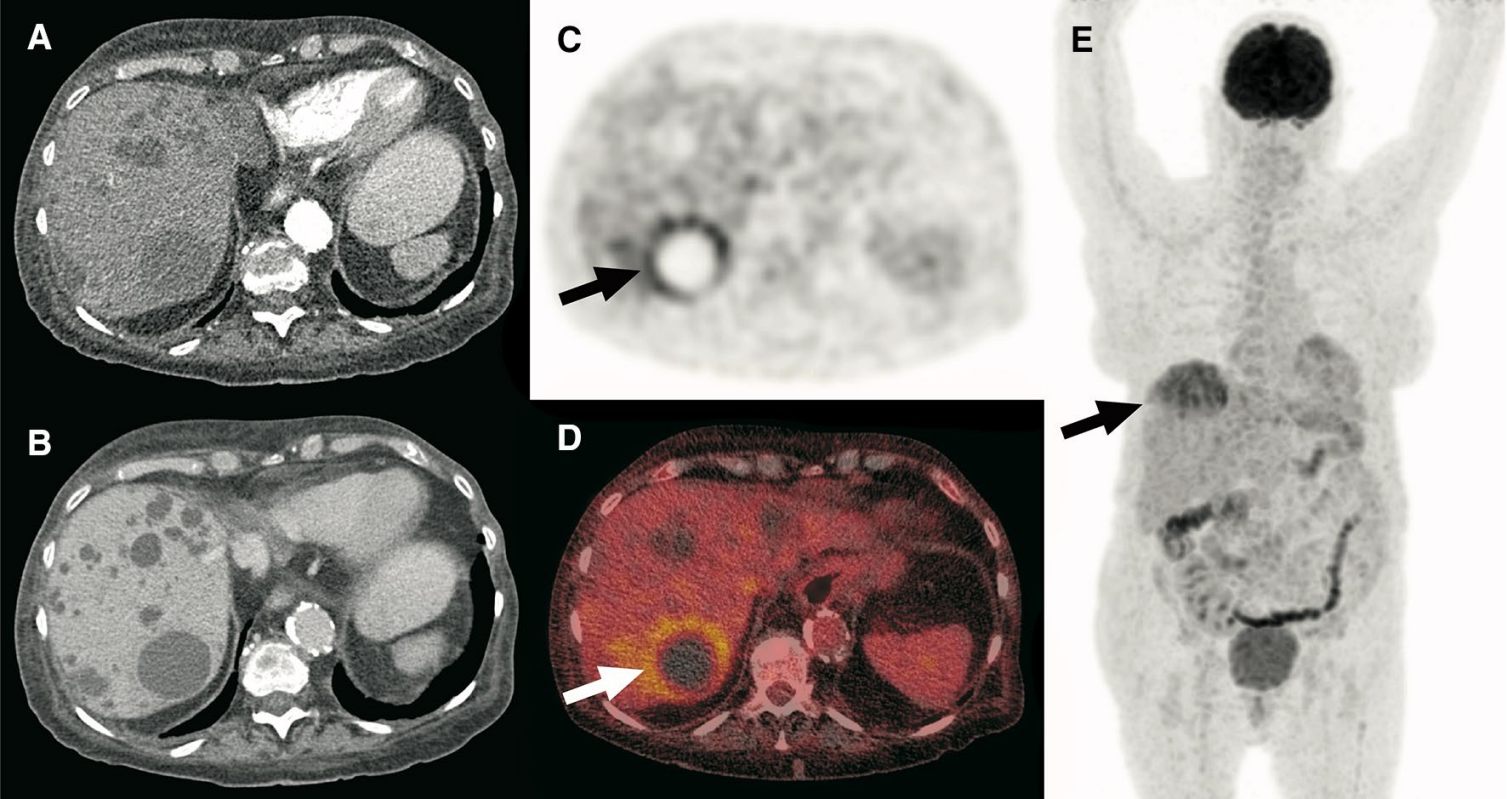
A 68-year-old woman with ADPKD presented with a feeling of general malaise that had been increasing over the course of 2 weeks. Nocturnal sweats were also present in the last week. There was no fever or abdominal pain. Blood tests showed a CRP level of 242 mg/L and a white blood cell count of 15.8 × 10^9^/L. Blood cultures were positive for *Escherichia coli* and urine cultures were positive for *Klebsiella pneumoniae*. The patient was admitted with a differential diagnosis of urosepsis or an infection of her abdominal aortic endograft. Despite intravenous treatment with cefuroxime for 4 days, infectious parameters remained high (CRP level of 228 mg/L). To find the source of infection, a contrast enhanced CT scan of the abdomen was ordered. Axial arterial phase (a) and portal-venous phase (b) full-dose CT scans showed multiple liver cysts that were otherwise unremarkable. Because CT failed to identify any site of infection, an FDG-PET/CT scan was ordered. Axial FDG-PET (c) fused FDG-PET/CT (d), and coronal maximum intensity projection FDG-PET (e) showed pathologic FDG uptake of the wall of a cyst in segment seven of the liver (arrows), in keeping with cyst infection. No pathologic FDG-avid foci were detected elsewhere. The antibiotic regimen was changed to ceftriaxone for better cyst penetration. CRP levels decreased to 49 mg/L in 4 days. The patient was sent home in good condition with a subsequent course of ciprofloxacin
